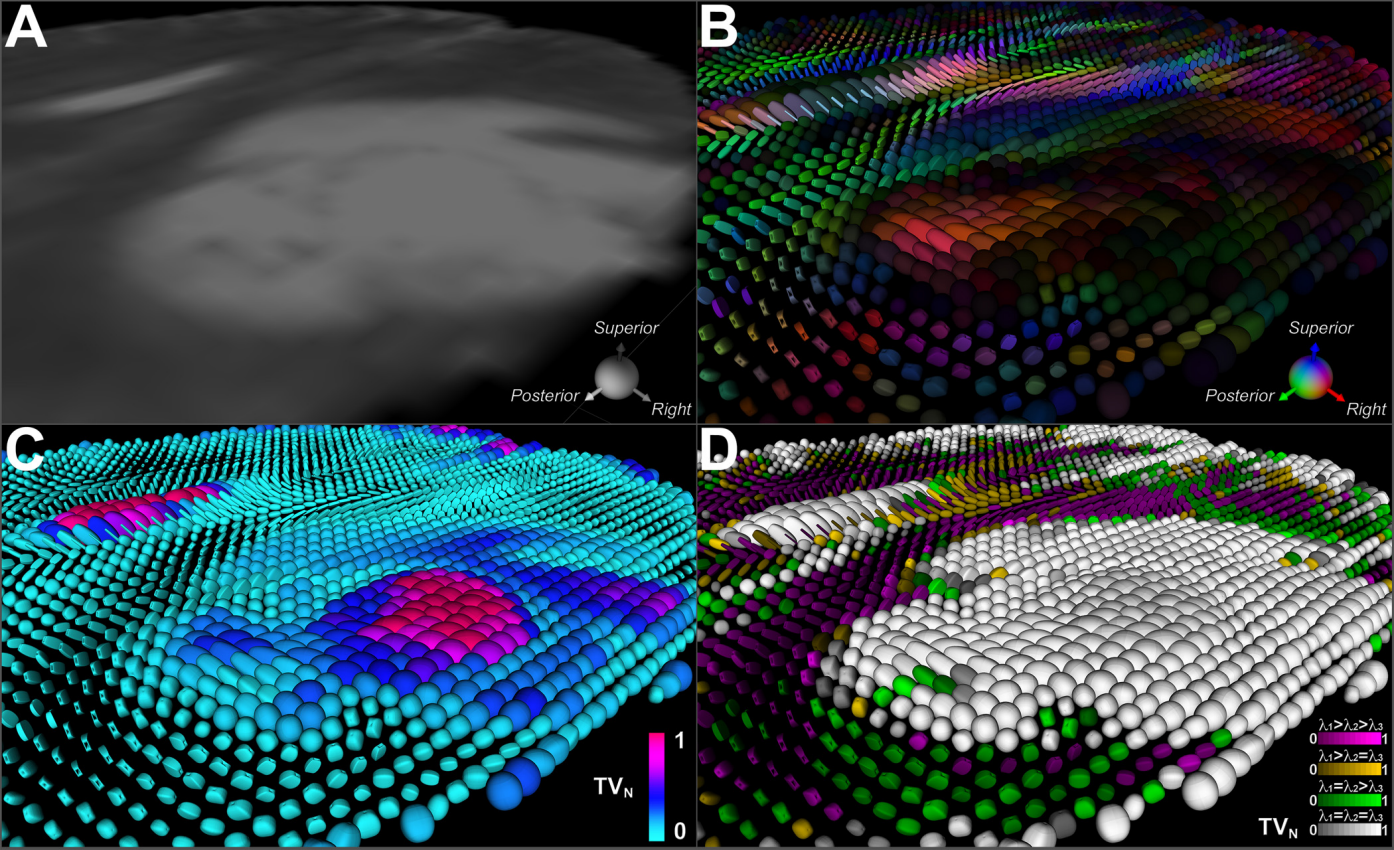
Supertoroidal field of a brain with a GBM color-coded with TV and eigenvalue configuration. (A) T2-weighted image of a mid-level axial cross-section of a brain with a GBM. (B) Supertoroidal glyph field color-coded by the orientation of the primary eigenvector and weighted by FA. (C) Supertoroidal glyph field color-coded with normalized TV index using a hot-to-cold color map, revealing the necrotic core and surrounding edema. (D) Supertoroidal glyphs encoded by the eigenvalue configuration with color saturation modulated by TV. These schemes (C, D) provide visual cues that enhance the identification of tissue architectural changes, as seen in the tumor-infiltrated tissue.
